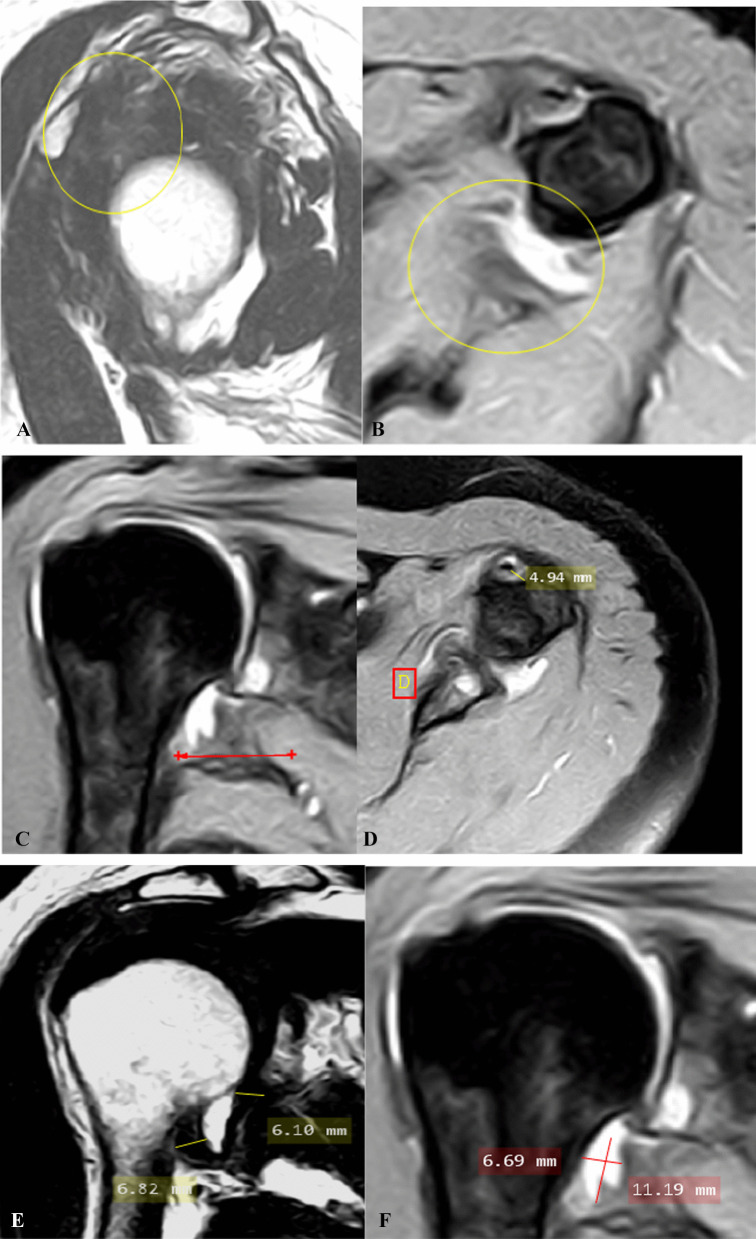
A Oblique sagittal PD- weighted MR image shows complete obliteration of the rotator interval with no observed fat planes or coracohumeral ligament (encircled area). B Oblique axial fat-suppressed PD-weighted MR image shows anterior and posterior extracapsular edema (encircled area). C Oblique coronal fat-suppressed PD-weighted MR image shows edema at humeral portion of axillary recess (arrow). D Oblique axial fat-suppressed PD weighted MR image shows effusion in long head biceps tendon sheath (arrow). E Oblique coronal T2-weighted MR image shows thickened joint capsule and measurement of maximum capsular thickness of glenoid and humeral portions of axillary recess with effusion at axillary recess. F Oblique coronal fat-suppressed PD-weighted MR image shows measurement of maximum height and width of axillary recess at the same plan
